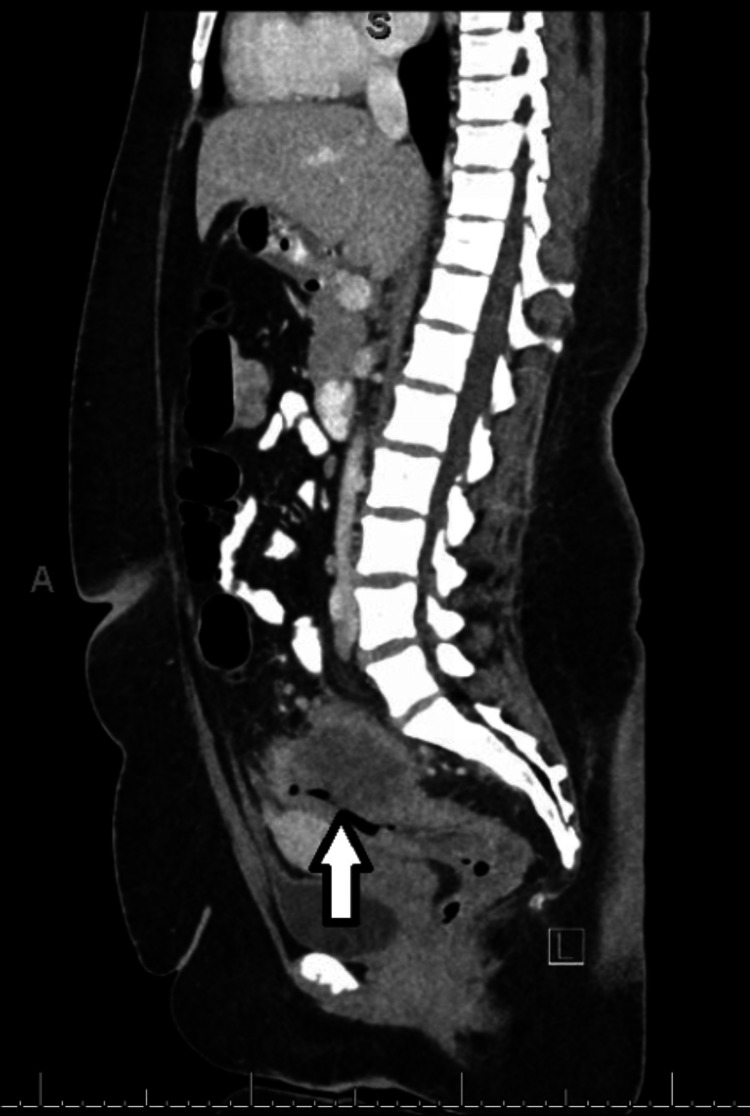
CT scan of the abdomen/pelvis demonstrating an inhomogeneous mass within the mid to left pelvis in vertical view
